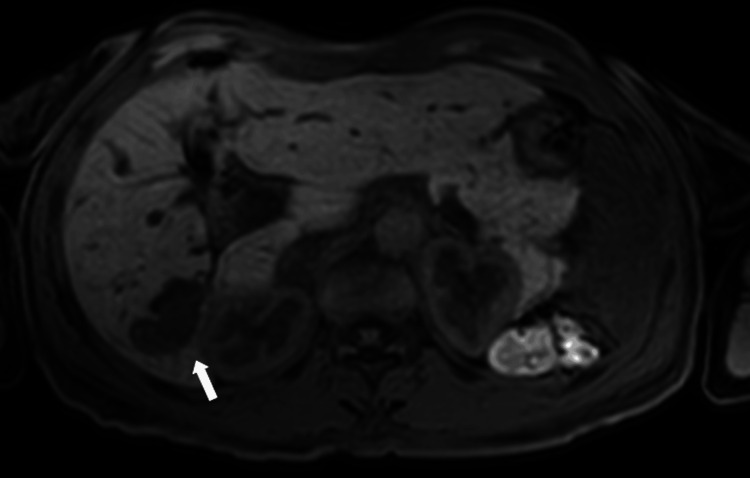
Axial T1-weighted MRI image at the level of the lesion Axial T1-weighted MRI showing the same intraductal lesion appearing hypointense relative to the liver parenchyma (white arrow).
